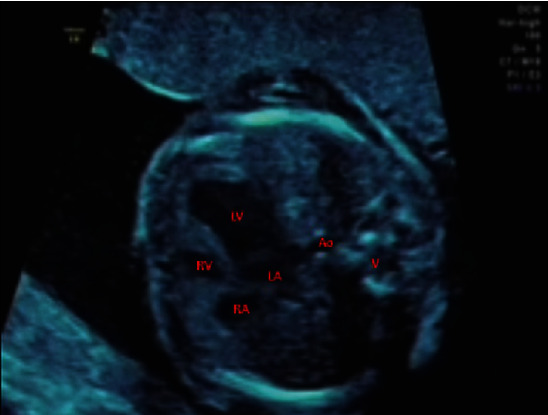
Apical four-chamber view showing the right ventricular cavity was moderately hypoplastic with the hypertrophic myocardium and intact ventricular septum.
